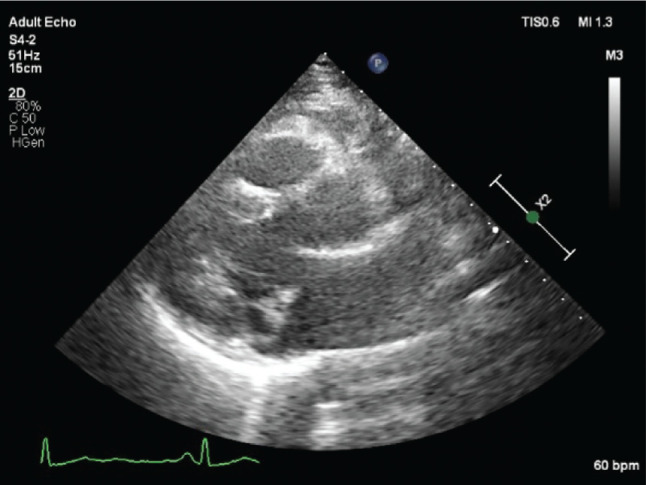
Case 1 echocardiogram showing bileaflet mitral valve prolapse in the context of Barlow’s disease.
